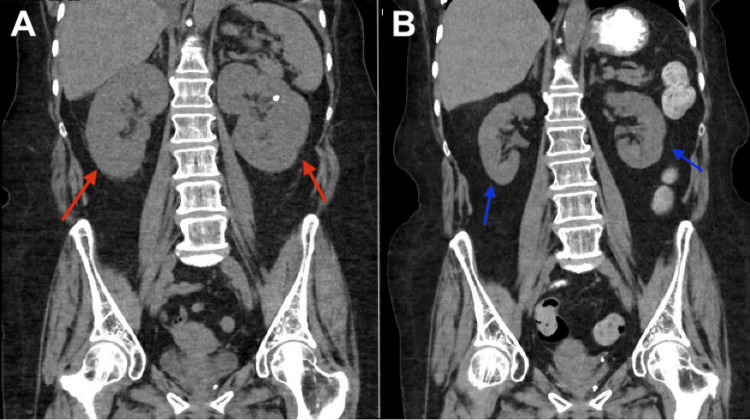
A: Computed tomography of the abdomen showing bilateral renal enlargement in a 66-year-old female diagnosed with PRL (red arrows). B: Improvement of bilateral renal enlargement on CT abdomen after treatment with six cycles of R-CHOP (blue arrows). PRL: primary renal lymphoma; R-CHOP: rituximab, cyclophosphamide, doxorubicin, vincristine, and prednisone
